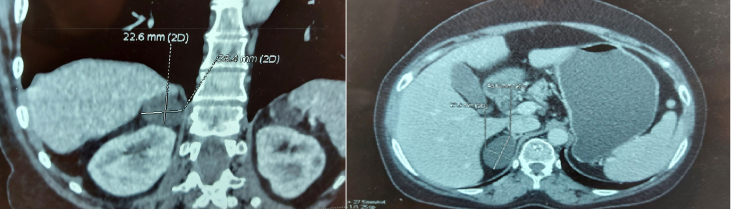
Computed tomography of a cystic right adrenal mass of oval shape and regular contours measuring 45*28*41mm.
